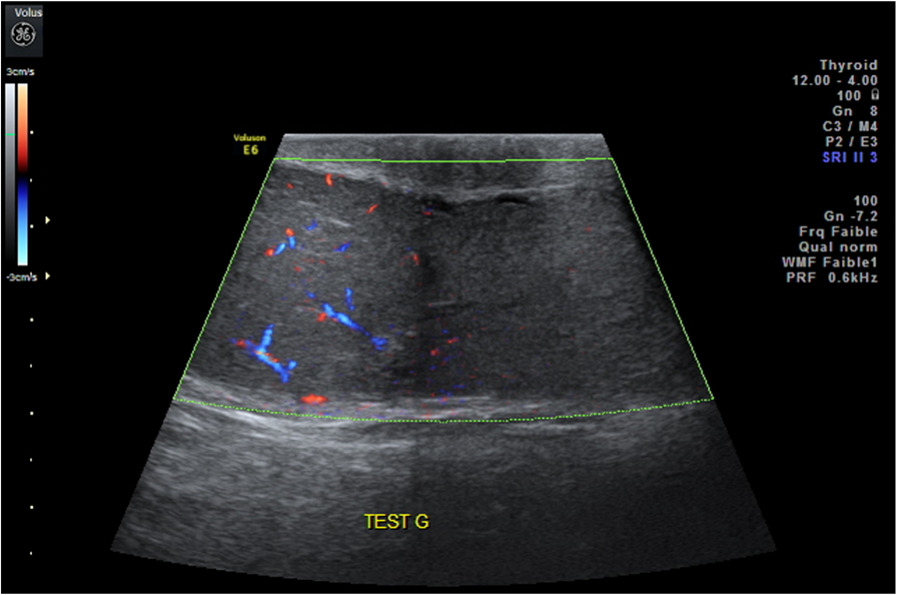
Scrotal ultrasound: poorly vascularized mass appended to the upper pole of the left testis
